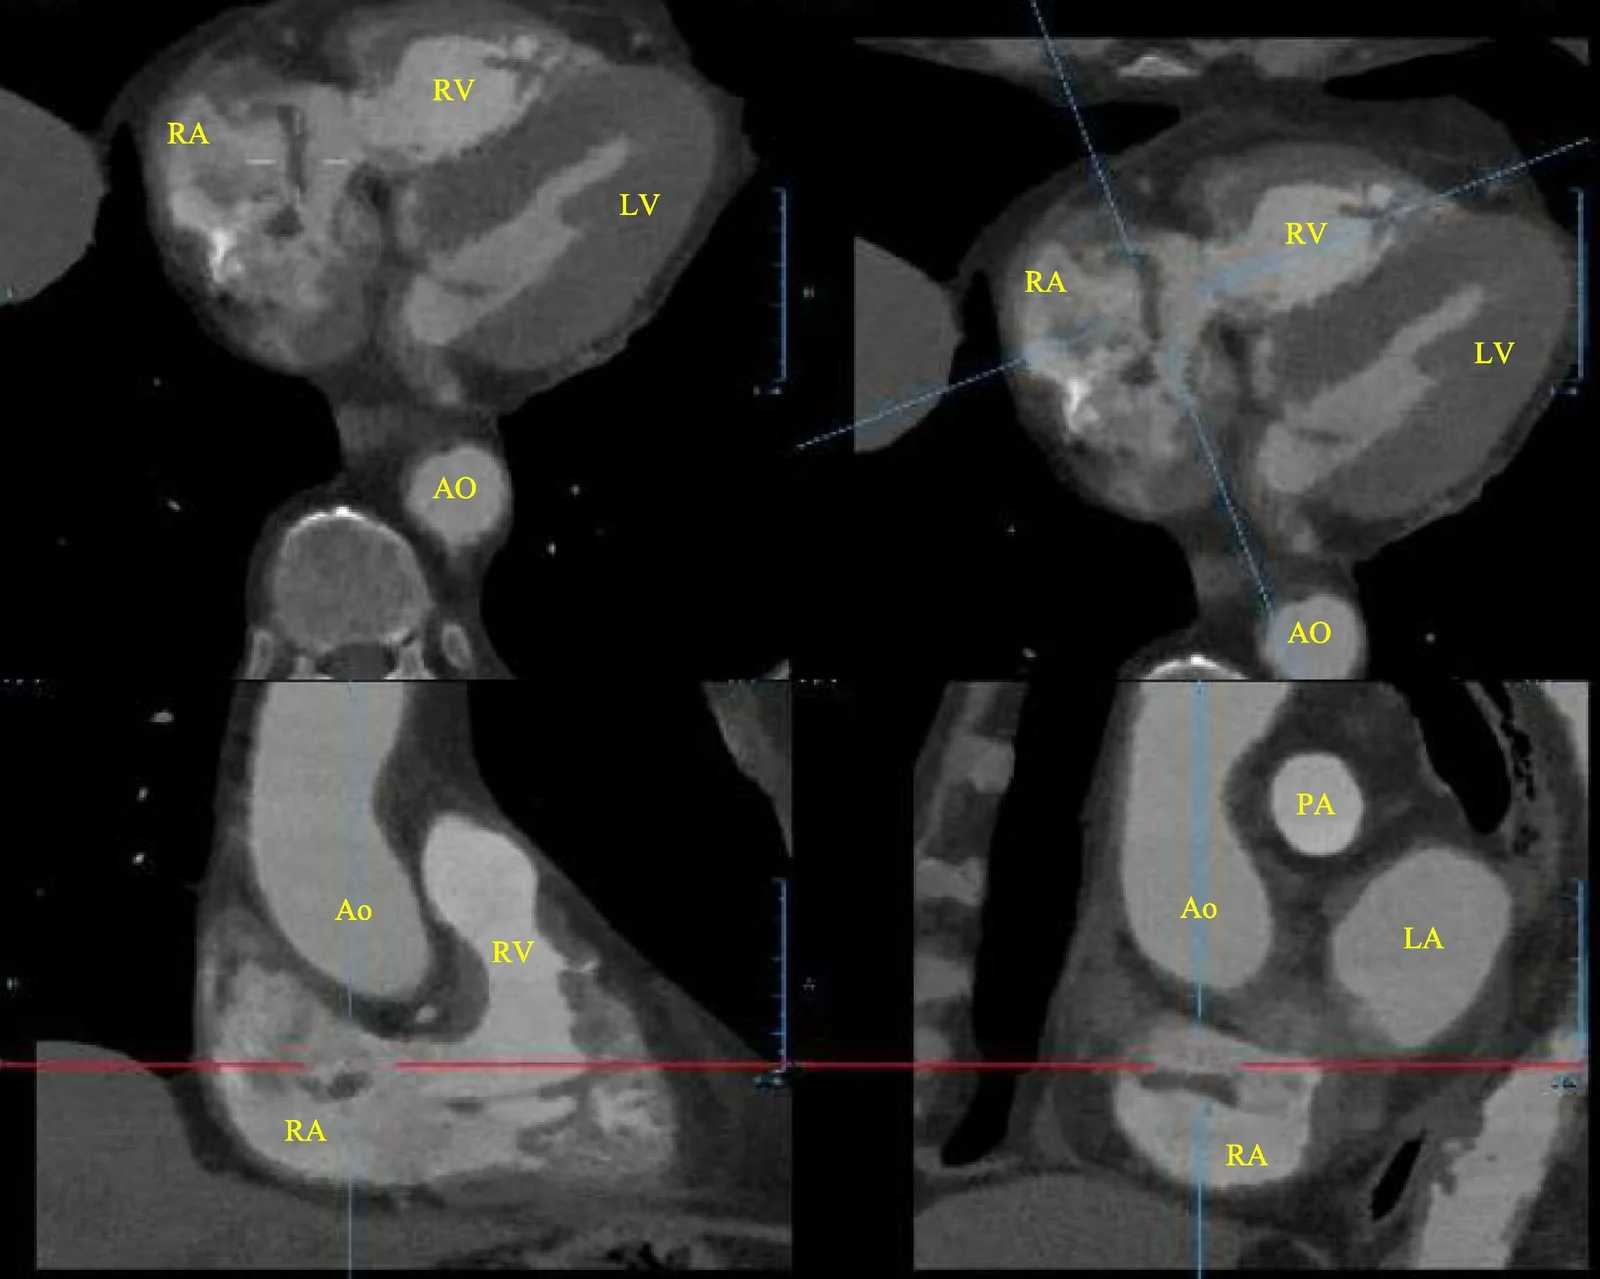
Photon-counting computed tomography multiplanar reconstruction demonstrating a mobile linear hypodense structure (measuring 39.8 mm) extending from the right atrial appendage base medially and dorsally towards the inferior vena cava, with diffuse irregular thickening of the right atrial appendage myocardium. Ao, aorta; LA, left atrium; LV, left ventricle; PA, pulmonary artery; RA, right atrium; RV, right ventricle.
